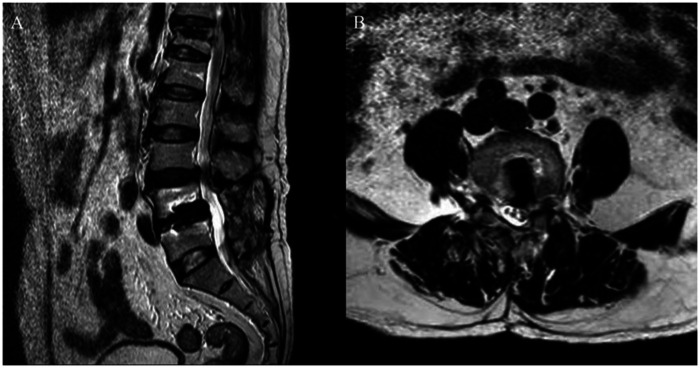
Six-month postoperative MRI showing no significant posterior cage displacement.
